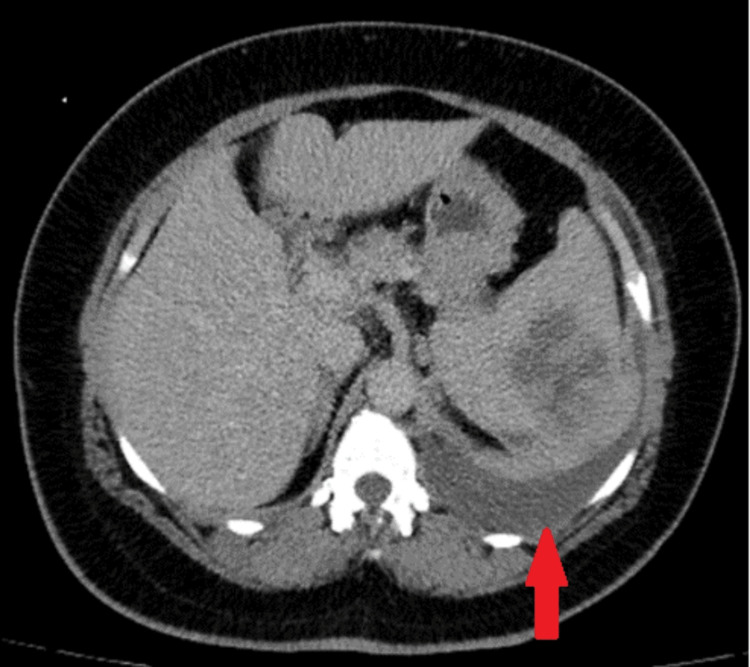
Follow-up CT abdomen with IV contrast in the axial view after three months showed rapid development of large left-sided pleural effusion (red arrow) with passive collapse consolidation of left lung lobes, with no signs of mediastinal lymph nodes.
